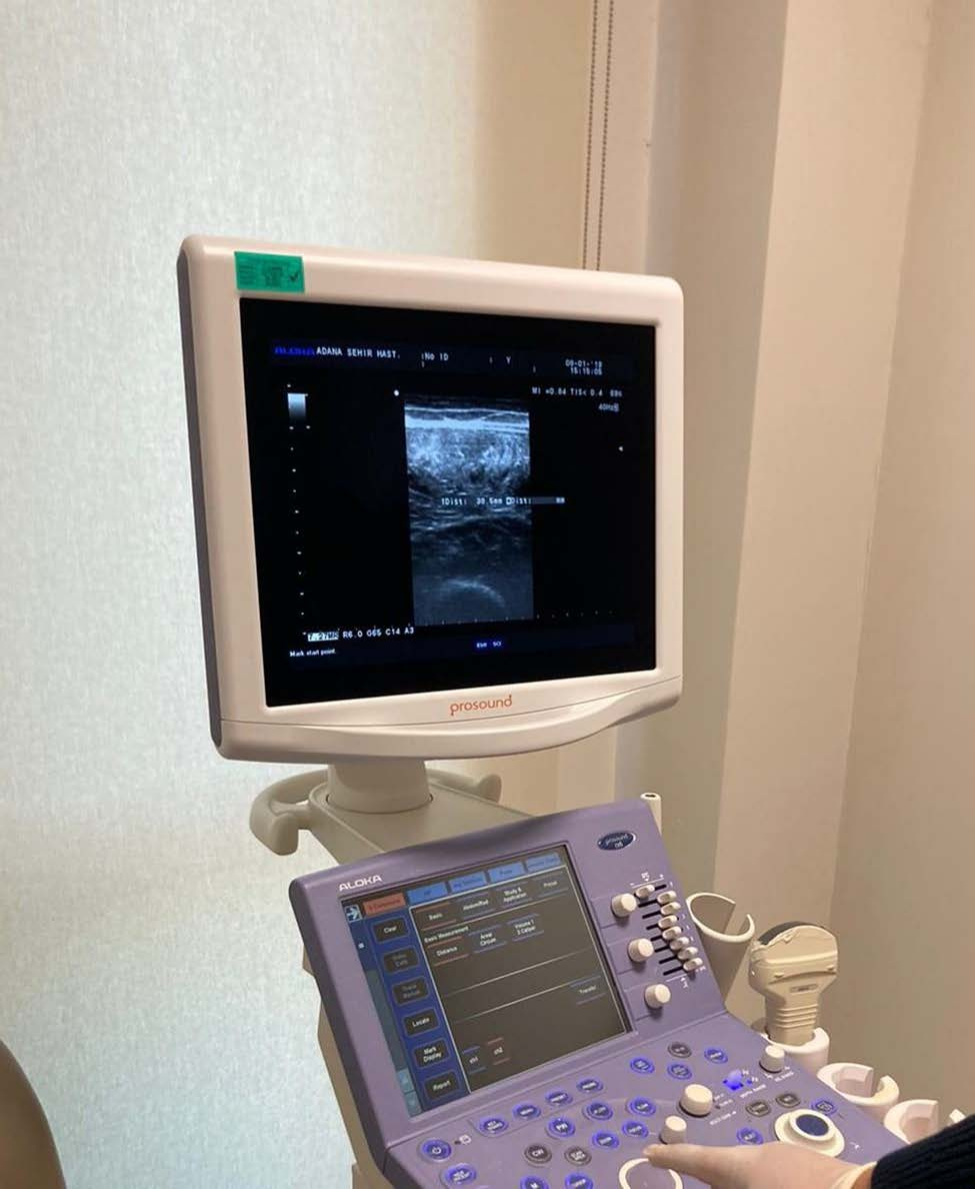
Ultrasonographic measurement of the Rectus Femoris muscle thickness.
